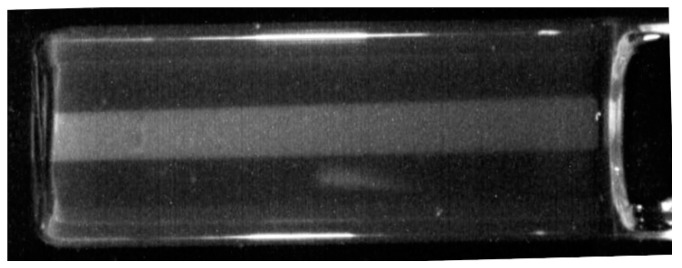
The image of a 5 × 5 mm^2^ 200 kVp X-ray beam propagating in a 60 mm length of RFG gel contained in a 20 × 20 mm^2^ cell. Optical artifacts occur at the glass/air sides of the cell and at the gel meniscus.
